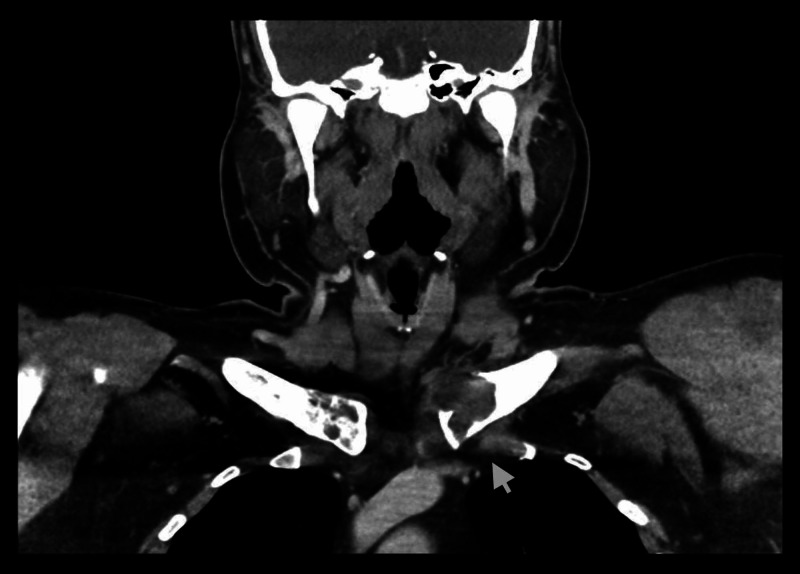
CT scan demonstrating a lytic lesion in left clavicular head
